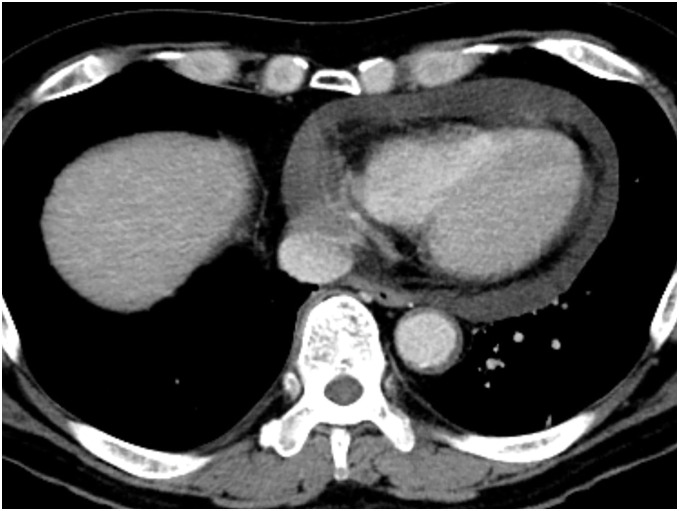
Enhanced CT image at the midnight of postoperative day 12 showing a moderate amount of pericardial effusion.
